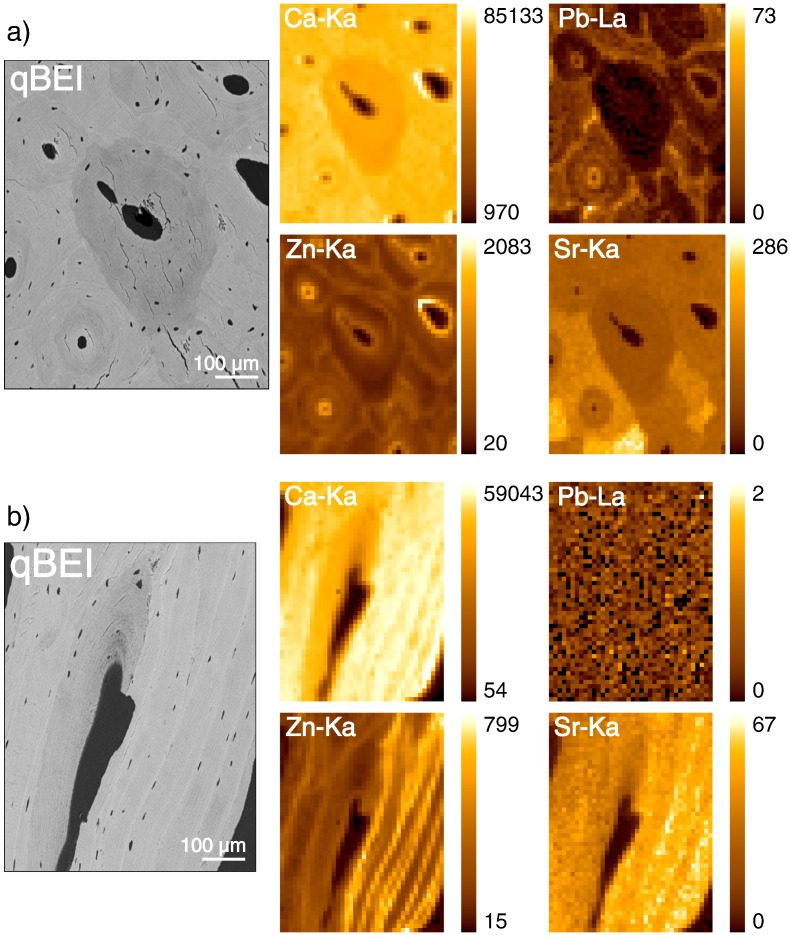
Examples of qBEI images with corresponding SR μ-XRF element maps of Ca, Zn, Pb, Sr: (a) osteonal bone region of the human femoral neck (b) cancellous bone region of the human femoral neck. The color-coded X-ray intensities are normalized to counts per second (cps), 100 mA Ring current and are scaled from minimum to maximum within each individual map. qBEI images show younger bone packets (less mineralized) as darker, and older bone packets (more mineralized) as brighter gray levels. Sample (b) exhibiting multiple parallel cement lines.
